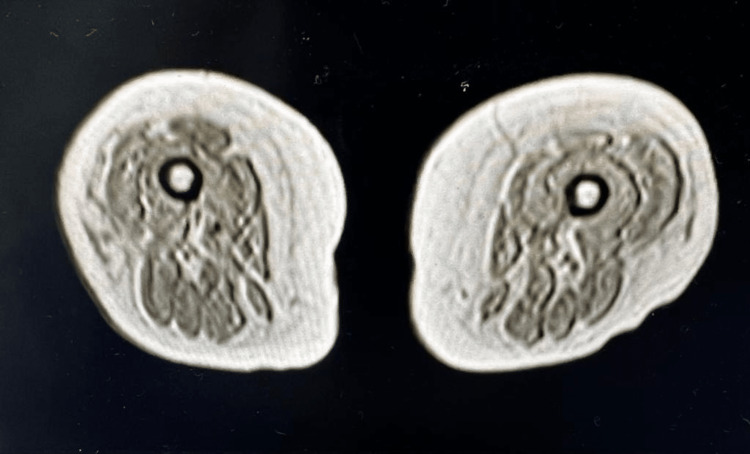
Magnetic resonance imaging showing symmetrical edema involving the proximal mid-thigh muscles.
